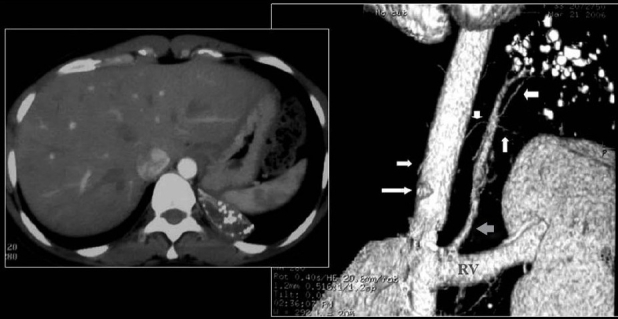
A rare calcified intralobar sequestration; the arterial supply and venous drainage is elegantly shown by the CT angiography (right) note that the arterial blood supply is arising from the celiac axis (white arrows) and venous drainage is via the left renal vein (gray arrow)
